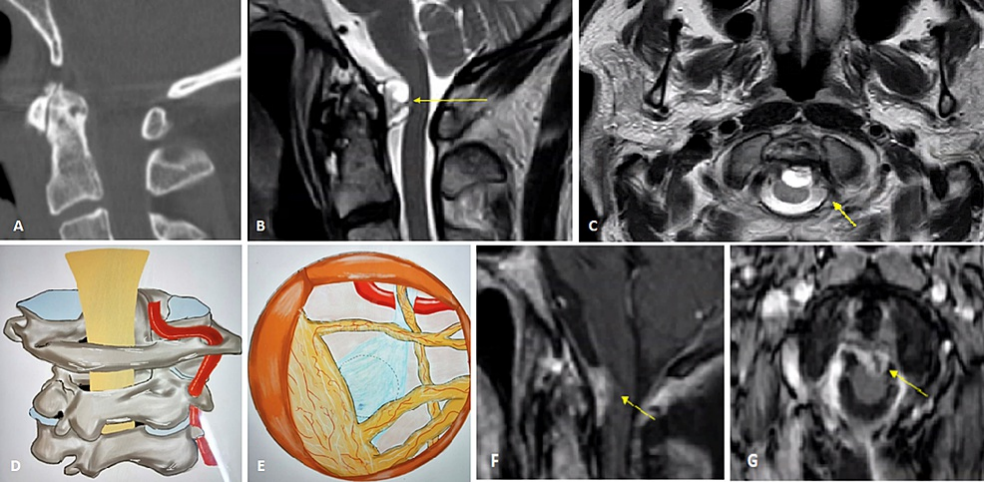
(A) CT neck demonstrating mild focal hyperostosis behind the dens. (B) Sagittal and axial (C) MRI of the head and neck demonstrating spinal stenosis at C1-2 from intradural extramedullary mass (IDEM) (arrow) impinging on the spinal cord at the level of dens. (D, E) Artist's rendering of the location of the retro-odontoid intradural mass. (F) Postoperative sagittal and axial (G) MRI of a resected cystic portion of the tumor and reduced mass effect (arrow) on the brainstem CT: computed tomography; MRI: magnetic resonance imaging
